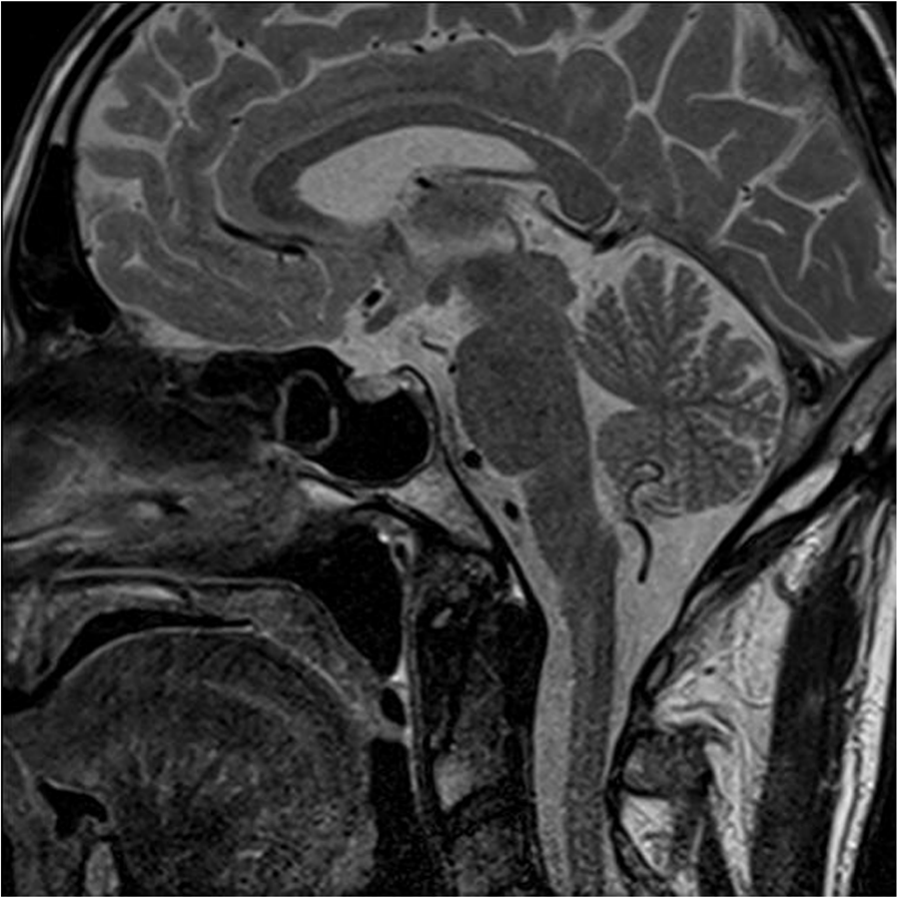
Post-contrast T2-weighted MR-image after several dosis of nivolumab reveals slight diffuse enlargement of the pituitary gland in the absence of micro- or macroadenoma
